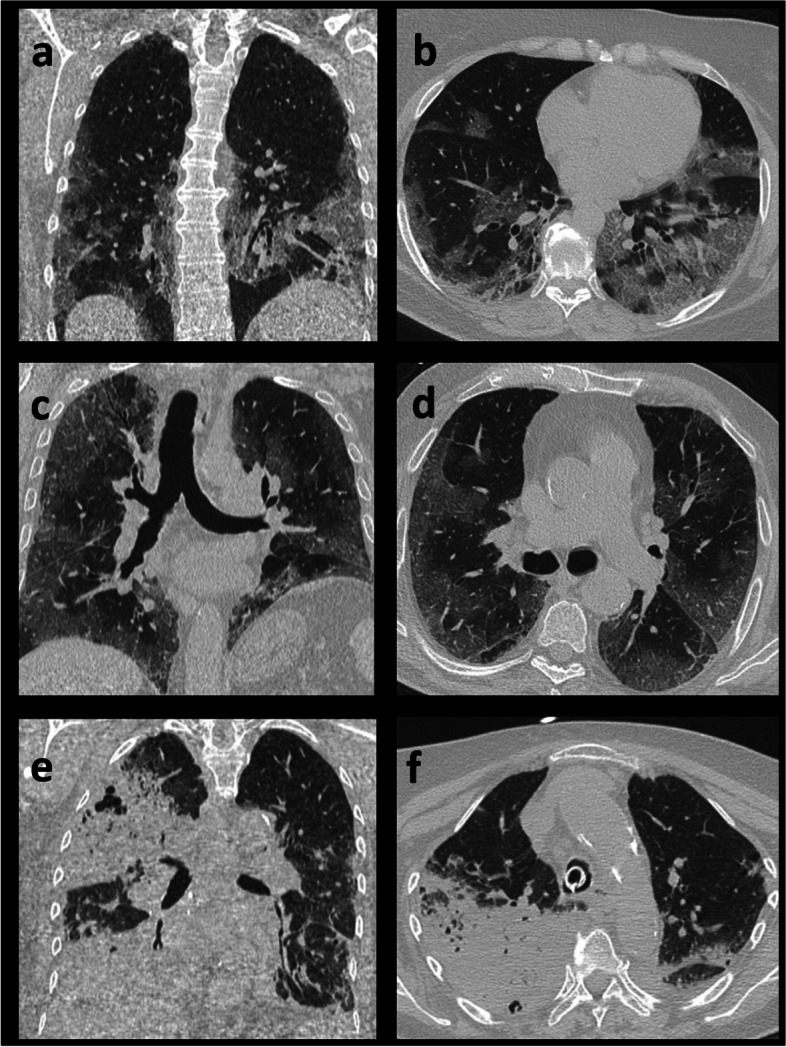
Chest CT scans in axial and coronal planes for: a, b crazy-paving pattern; c, d extensive lung involvement with predominantly ground-glass opacities; e, f consolidation with air bronchogram of the right middle lobe extending to the right superior lobe
